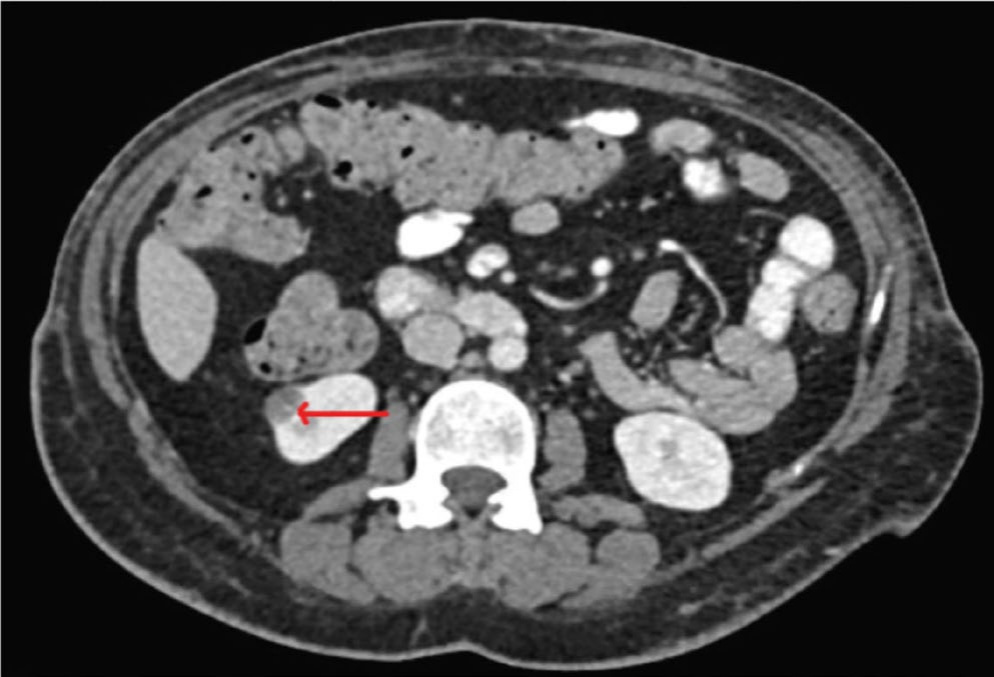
A CT scan of the abdomen with contrast showing a right renal complex cystic lesion.
